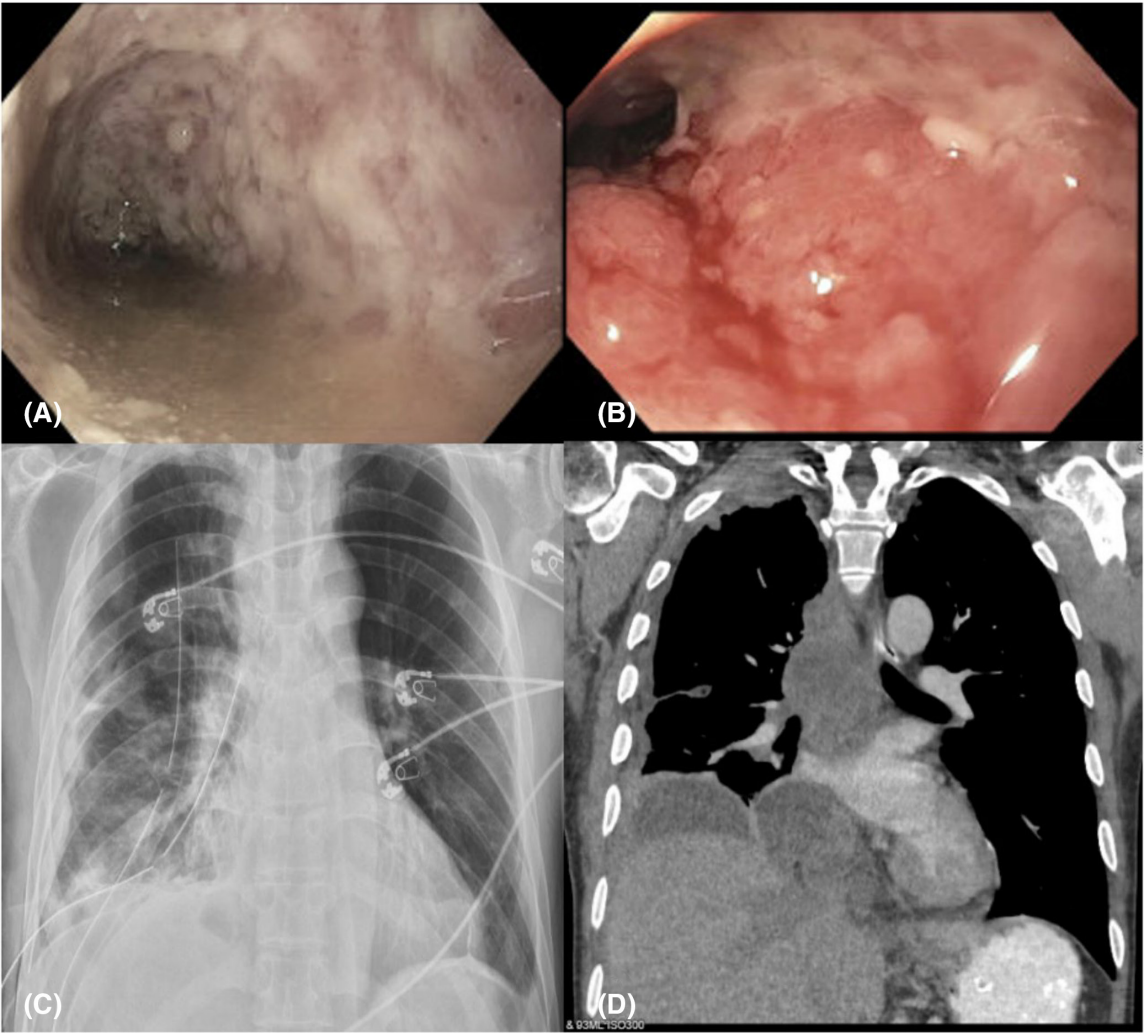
Imaging shows pseudomembranes suggesting *Clostridium difficile* colitis and empyema with cultured *Nocardia*. (A) Flexible sigmoidoscopy shows pseudomembranes in the sigmoid colon consistent with *C*. *difficile* colitis. (B) Flexible sigmoidoscopy shows ulcerations and pseudomembranes in the sigmoid colon. (C, D) Chest X‐ray and CT show worsening large right‐sided pleural effusion 48 h after initiation of upadacitinib.
